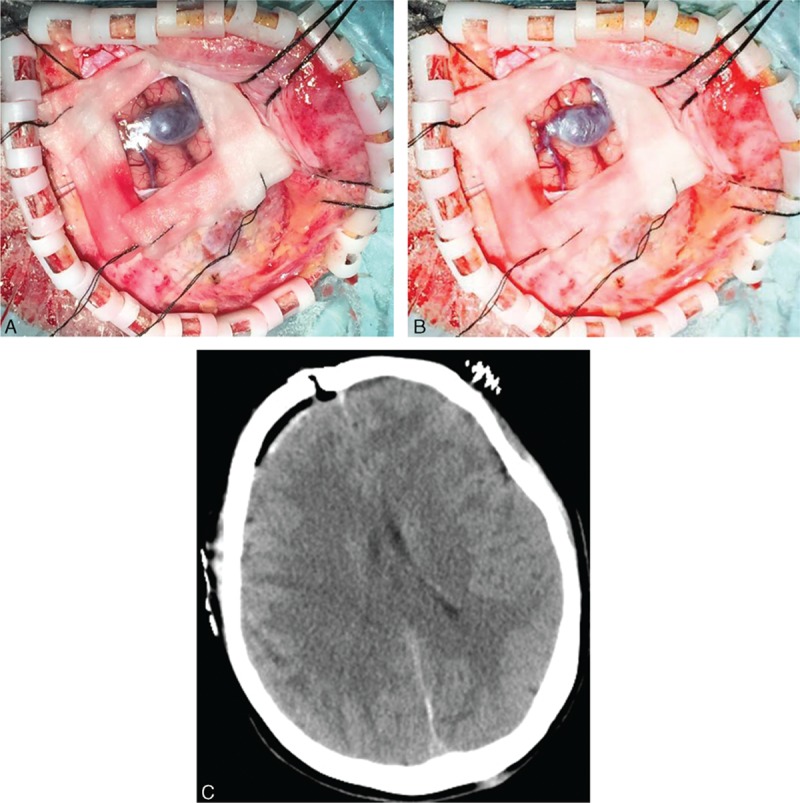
Intraoperative exposure of the isolate cerebral varix and postoperative CT image. A focal dilatation of the convexity vein was found. It has the filling stage (A) and the loose stage (B), which prompts the press on the surrounding cortex, dura and the inner table of skull. No signs of venous congestion or brain tissue edema were observed in the CT image 24 h after the surgery (C). CT, computed tomography.
